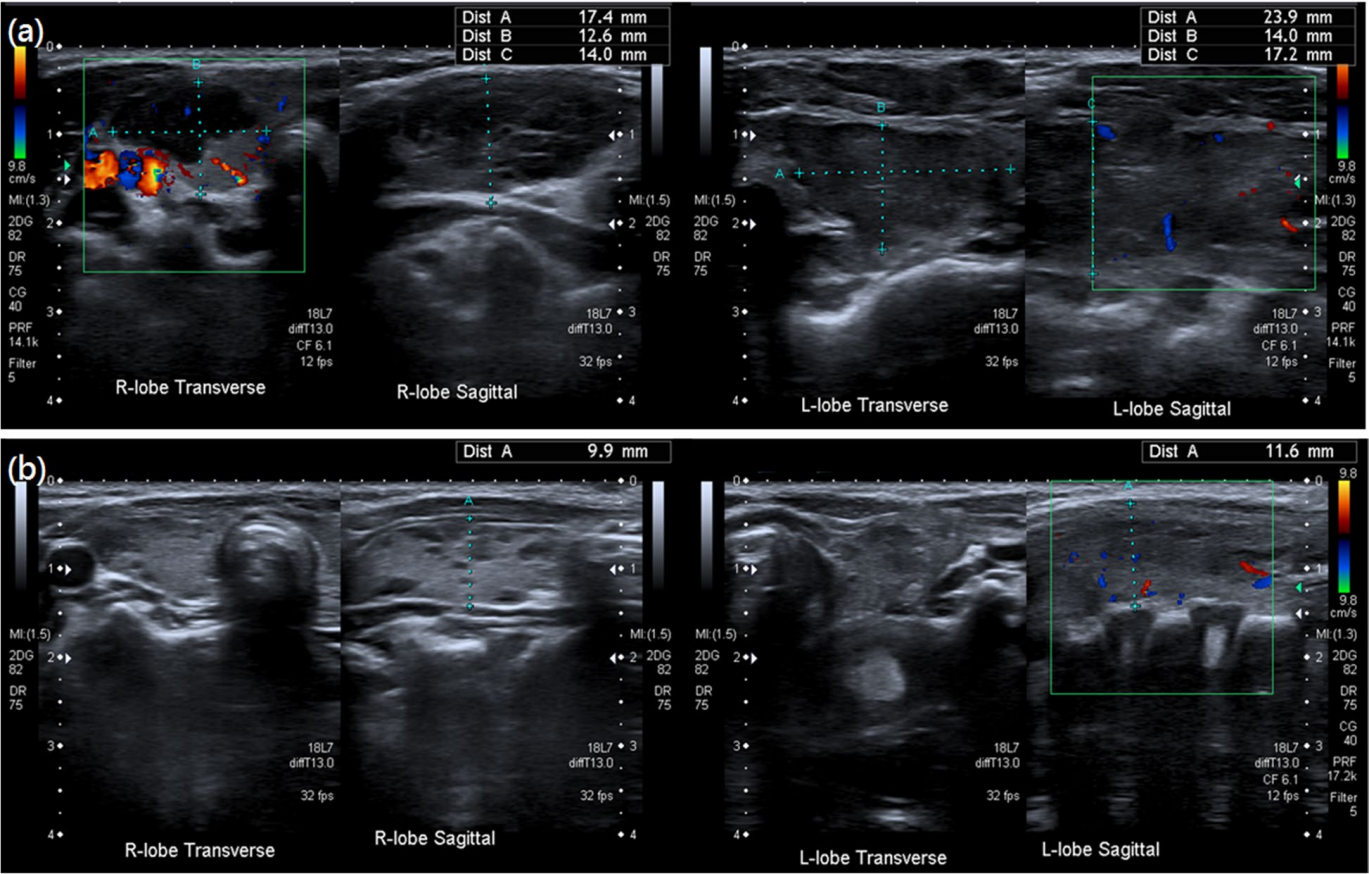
Follow-up sonography reveals notable improvement in the hypoechoic nodules and a restoration of the size of the thyroid lobes. a Before treatment; b After treatment
